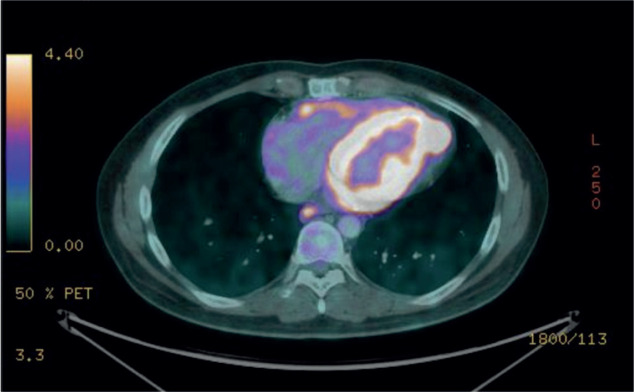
FDG PET scan with increase in both the size and metabolic tumor volume of the pericardial lesions which have heterogeneous density, predominantly hypodense, with persistent abnormal radiotracer uptake and a SULmax of 14.5 and VTM of 77.3.
